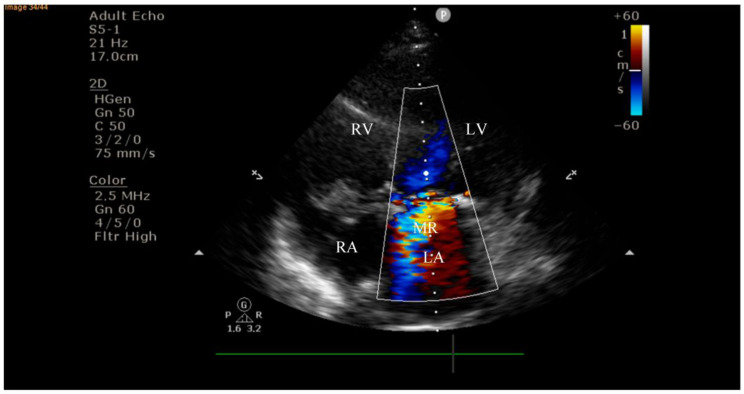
Apical four-chamber view on admission demonstrating severe mitral regurgitation with a large eccentric regurgitant jet directed into the left atrium; LV—left ventricle; RV—right ventricle; LA—left atrium; RA—right atrium; MR—mitral regurgitation jet.
